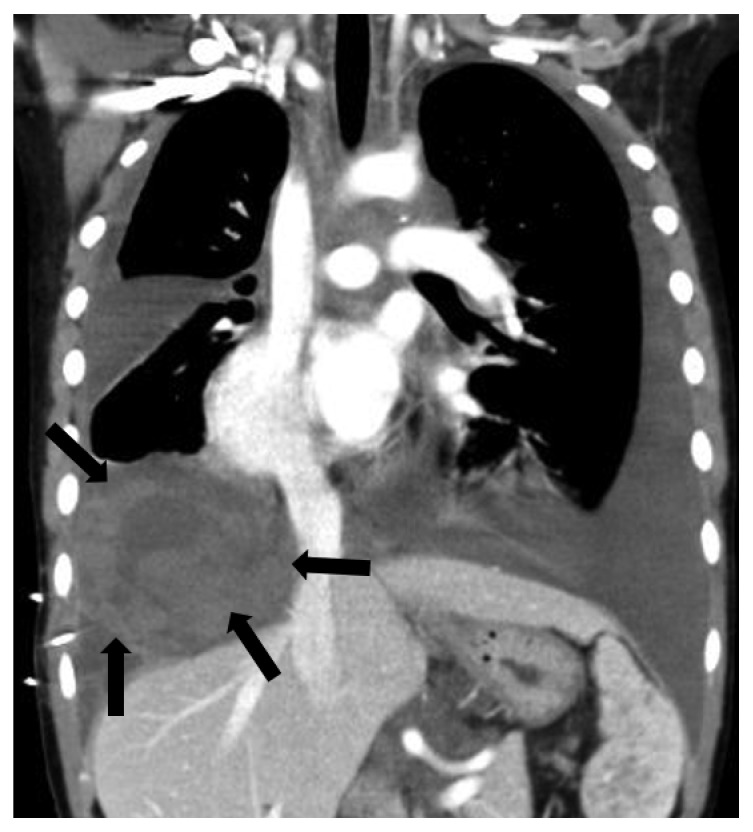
A chest contrast-enhanced computed tomography scan showing massive pleural effusion in both lungs. The black arrow shows a high-density hematoma in the right lung.
